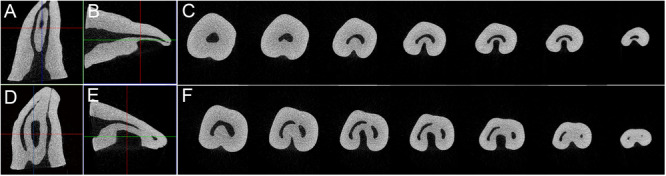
Representative two‐dimensional micro‐CT images of C‐shaped canal replicas demonstrating reduced canal wall thickness beneath radicular grooves. (A–C) A replica with continuous C‐shaped canal configuration (Melton's Category I) along the entire root length: (A) coronal section, (B) sagittal section, and (C) serial axial sections at various root levels. (D–F) A replica exhibiting discontinuous C‐shaped canal configuration (Melton's Category II and III): (D) coronal section, (E) sagittal section, and (F) serial axial sections at different root levels.
